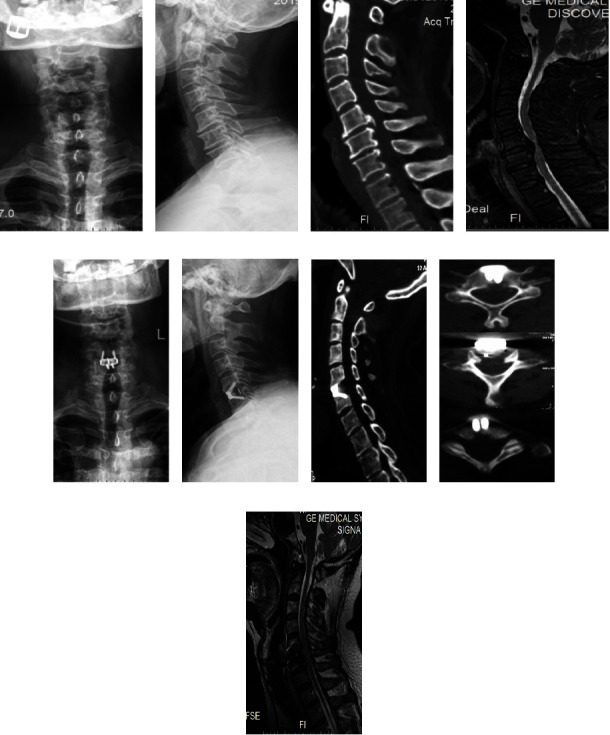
56-year-old man with CSM treated with ACDF using piezosurgery: (a, b) preoperative anteroposterior and lateral X-ray images showed the presence of large osteophytes in the C_5/6_ vertebral bodies, (c) preoperative sagittal CT examination showed C_5/6_ intervertebral disc herniation with calcification, (d) preoperative sagittal MRI revealed the C_5/6_ disc herniation that compressed the spinal cord, (e, f) postoperative anteroposterior and lateral X-ray examination showed that the plate implants were in good position and the posterior vertebral osteophytes had disappeared, (g, h) postoperative sagittal CT showed that C_5/6_ posterior osteophytes were removed completely, and the cervical canal area was significantly increased, and (i) postoperative sagittal T2-weighted MRI showed relief of C_5/6_ spinal cord compression.
